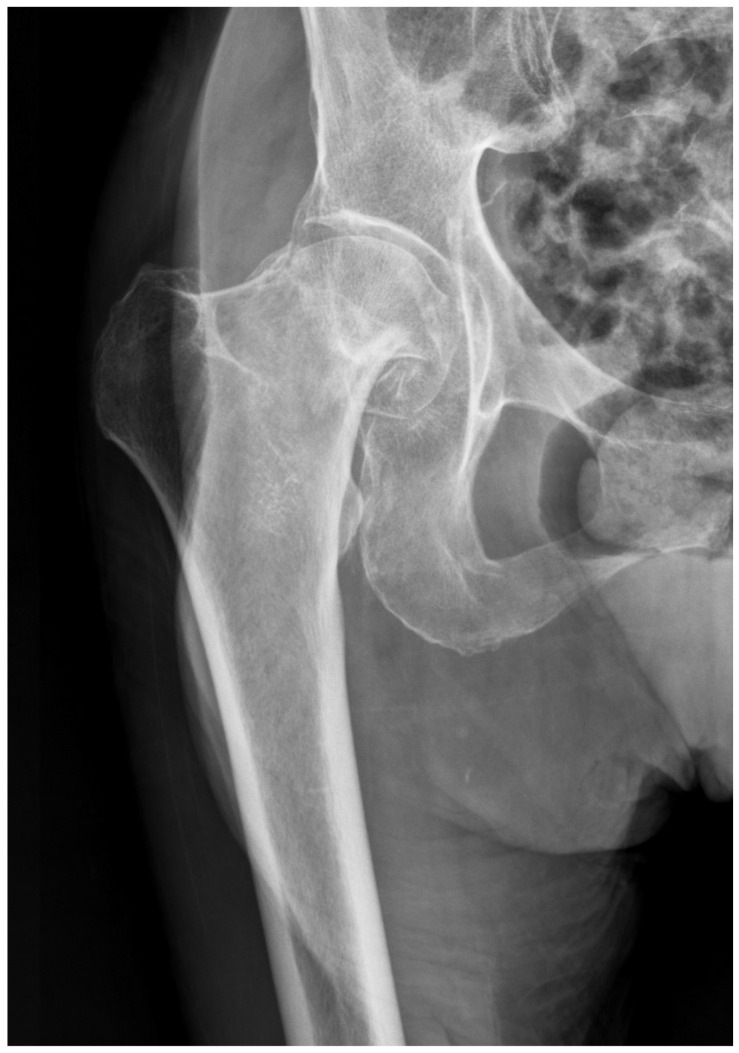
Femoral neck fracture as seen on pelvic radiograph.
